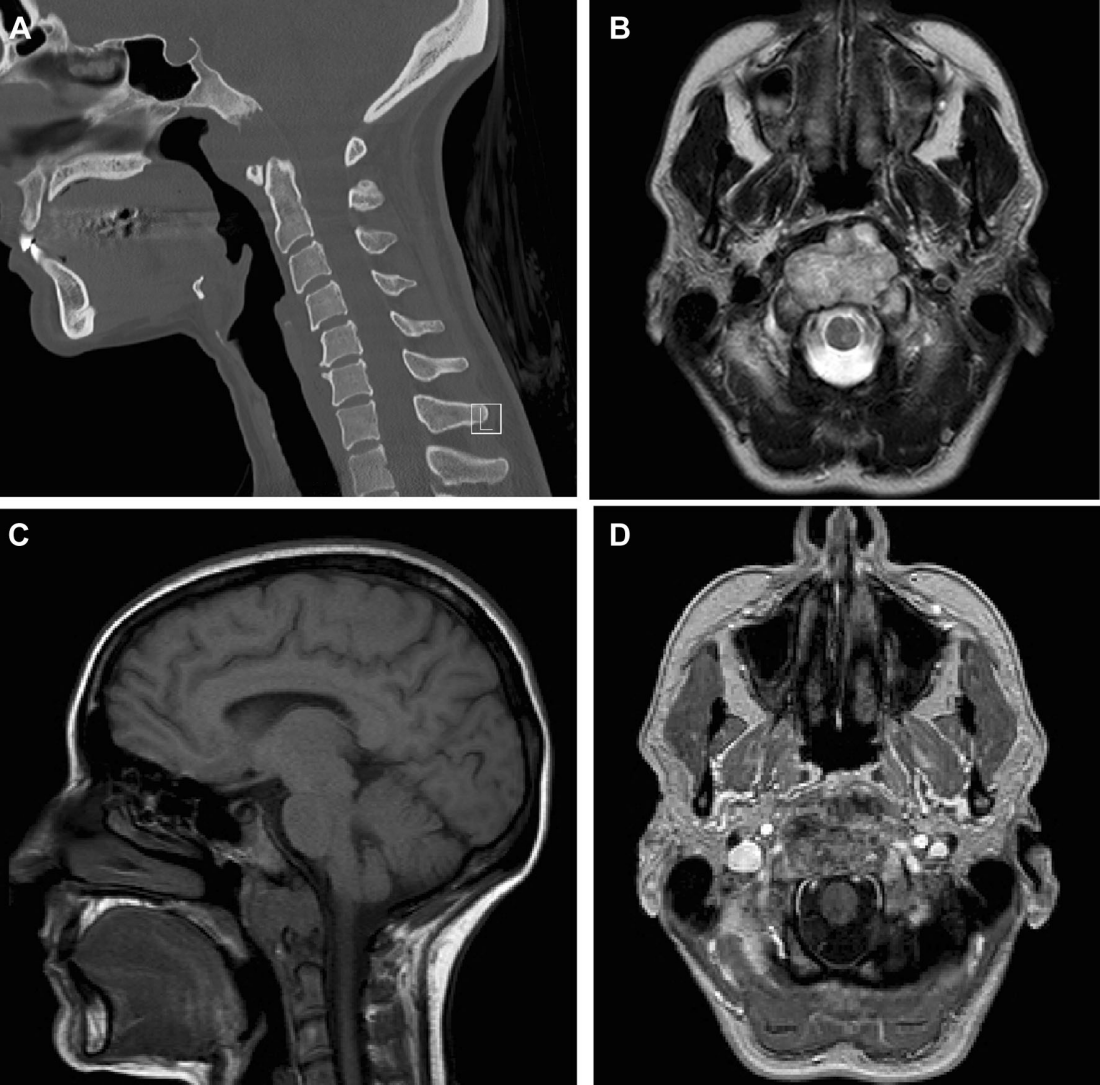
Chordoma. Imaging of a chordoma at the craniocervical junction. Computed tomography (a) shows lysis of the lower clivus. Axial T2-weighted imaging (b) shows a heterogenous, mostly hyperintense mass at the midline of the lower clivus. There is anterior extension to the oropharynx. The mass is hypointense on T1-weighted imaging (c) with heterogenous contrast enhancement (d). Pathologic examination confirmed the diagnosis of chordoma
